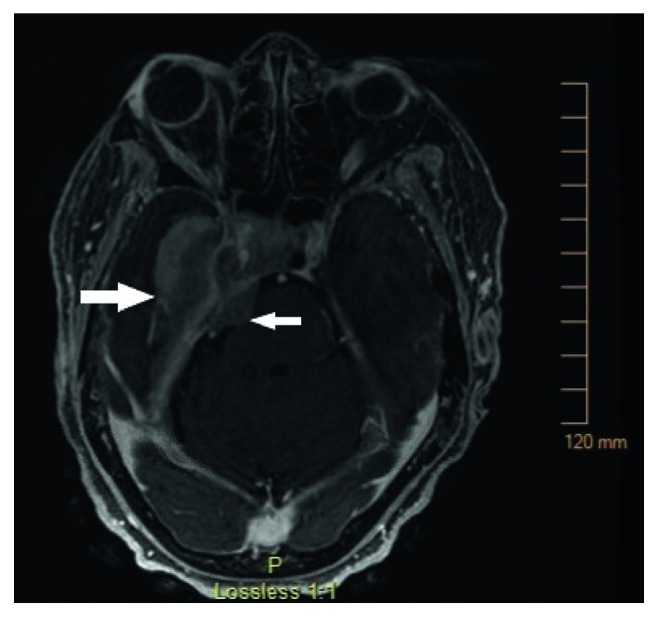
T1-weighted magnetic resonance imaging (MRI) of the head demonstrating abnormal signal along the dural surface of the right medial petrous region (smaller arrow) and paraclinoid region as well as sphenoid bone and cavernous sinus with upper sphenoid tumor extension (larger arrow), also extending to the foramen ovale and into the upper margins of the right infratemporal fossa.
